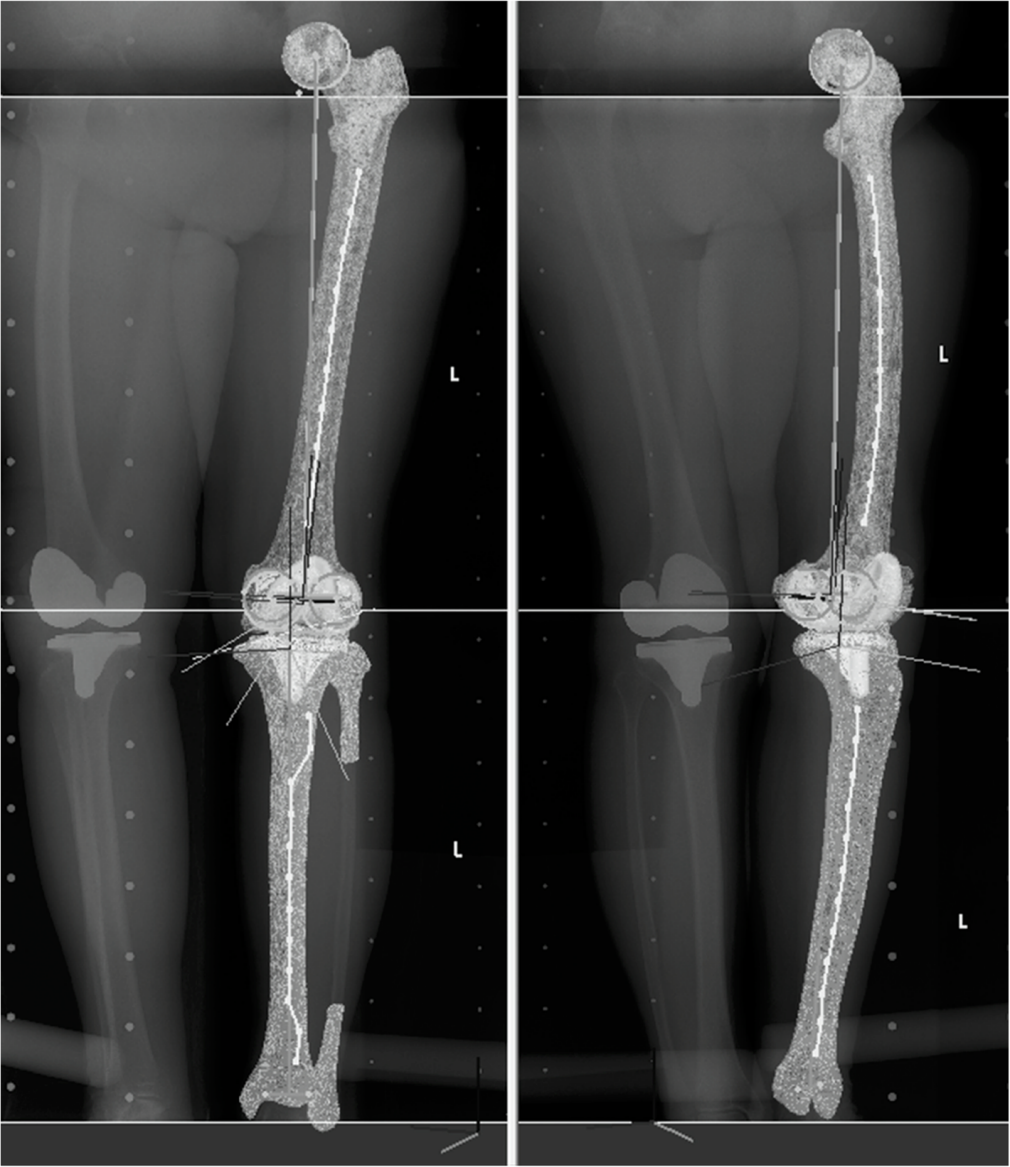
The three-dimensional (3D) post-operative evaluation of component position was performed using the same co-ordinates’ system as pre-operative planning. The information of the three-dimensional (3D) component position was obtained using 2D-to-3D image-matching technique for matching pre-operative computed tomography (CT) bone model and implant computer-aided design (CAD) model with post-operative biplanar CRs
